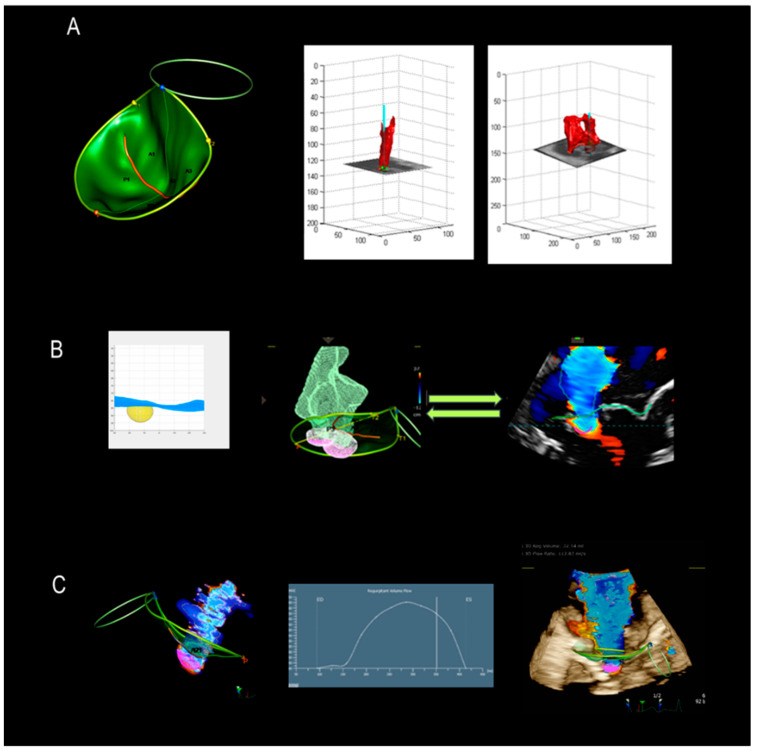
(A) The first step is the automated reconstruction of the volume rendering surface of the MV throughout the entire systole. The color flow is displayed in every frame and shape; the number and position of the orifice/s are assessed using the intersection of CF Doppler and MV surface rendering. (B) The second step is that the algorithm fills the orifice(s) with hundreds of tiny pinholes, and then generates a model of the convergence zone, displaying it as a purple mesh. This shape is compared to CF Doppler jet(s) in all systolic frames. The system can be reshaped by adding/removing pinholes to create a perfect match with 3D color Doppler. (C) The left panel shows Auto CFQ; in the middle panel, the graft shows the variation of regurgitant flow during systole; and the right panel shows the fusion of the 3D TEE image, surface rendering, and Auto CFQ.
